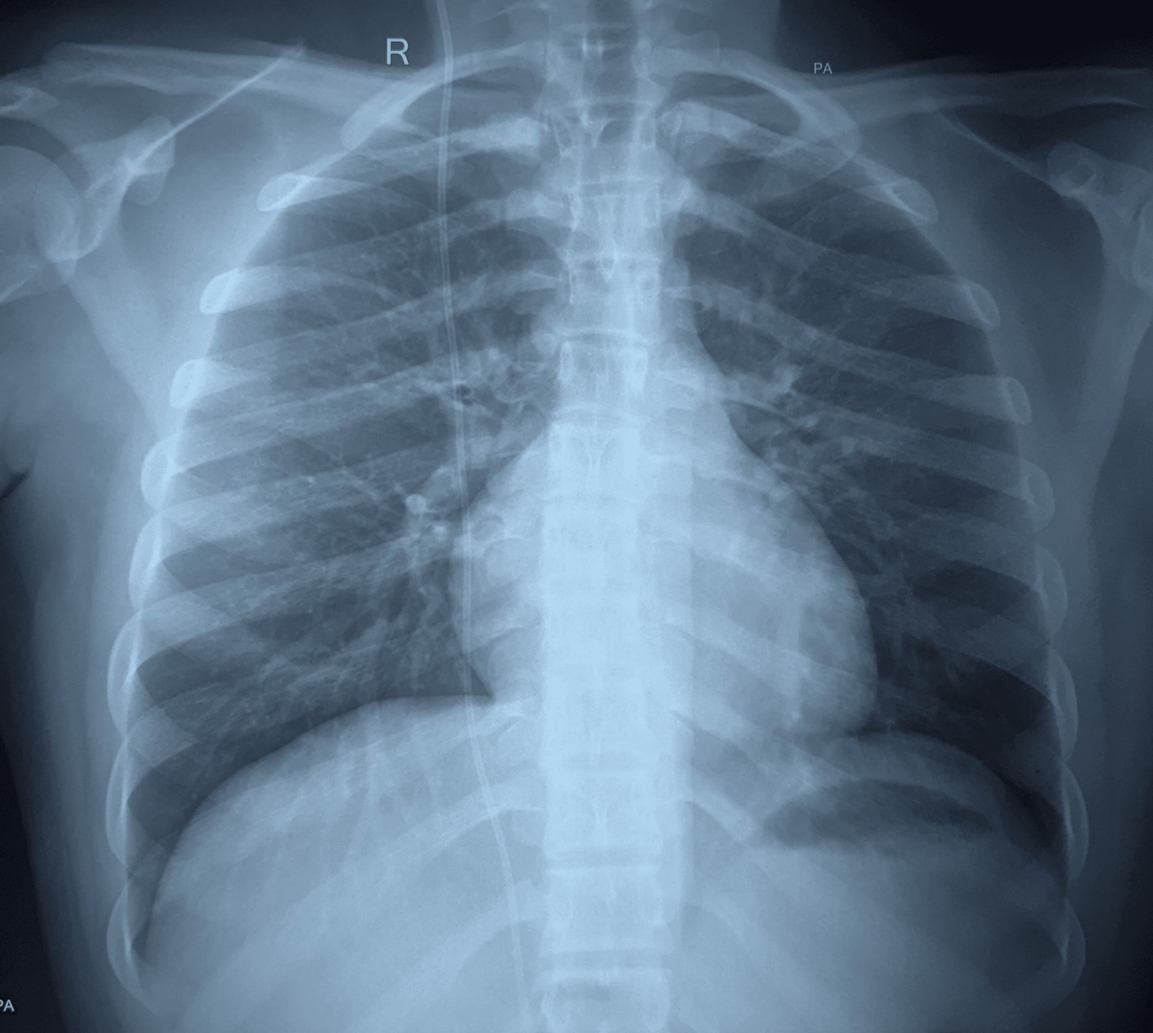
Immediate postoperative X-ray of chest (PA view) showing intact ventriculoperitoneal (VP) shunt position
